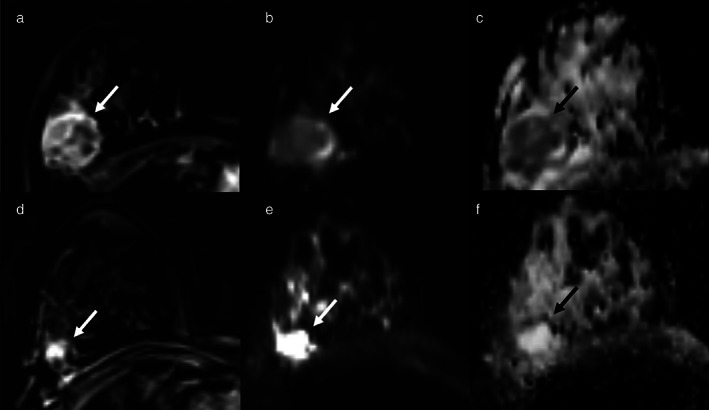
Example of early assessment of the response to neoadjuvant chemotherapy (NAC) using diffusion weighted imaging (DWI). examinations; (a–c) = pre‐NAC examinations; (d–f) = early assessment examination after two cycles of cytotoxic NAC. (a and d) = dynamic post‐contrast images; (b and e) = DWI images; (c and f) = ADC maps. A 37‐year‐old patient with a G3, triple negative invasive ductal carcinoma of the right breast (white and black arrows). Early assessment showed a reduction of tumor size along with increase of signal intensity on ADC maps (c) compared to the pre‐treatment examination (f). Pathology after surgical resection revealed pathological complete response. Reprinted under a Creative Commons (CC BY 4.0) license from: Romeo V, Accardo G, Perillo T, Basso L, Garbino N, Nicolai E, Maurea S, Salvatore M. Assessment and Prediction of Response to Neoadjuvant Chemotherapy in Breast Cancer: A Comparison of Imaging Modalities and Future Perspectives. Cancers (Basel). 2021;13(14):3521.
